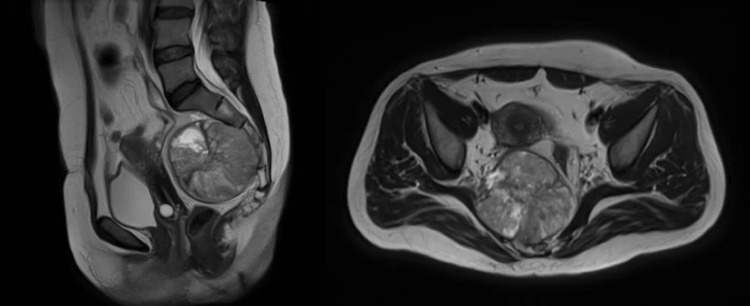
Magnetic resonance imaging. (A) Axial view and (B) sagittal view.
